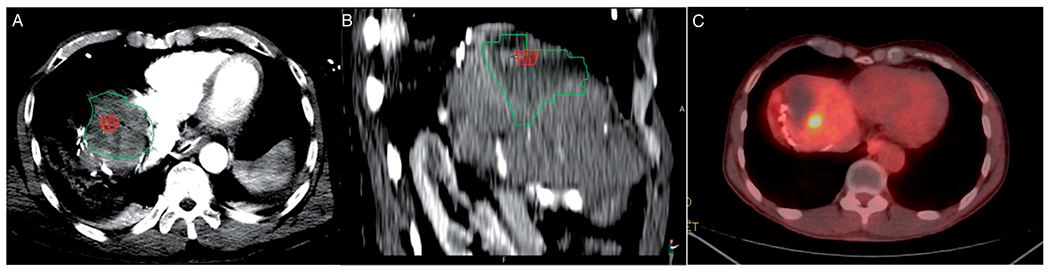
Colorectal metastasis (red) ablation in the liver dome. Ablation zone (green) with adequate margins in the axial plane (A), but suboptimal margins in sagittal plane examination (B). Local tumor progression is evident on a 10-month PET/CT follow-up scan (C).
